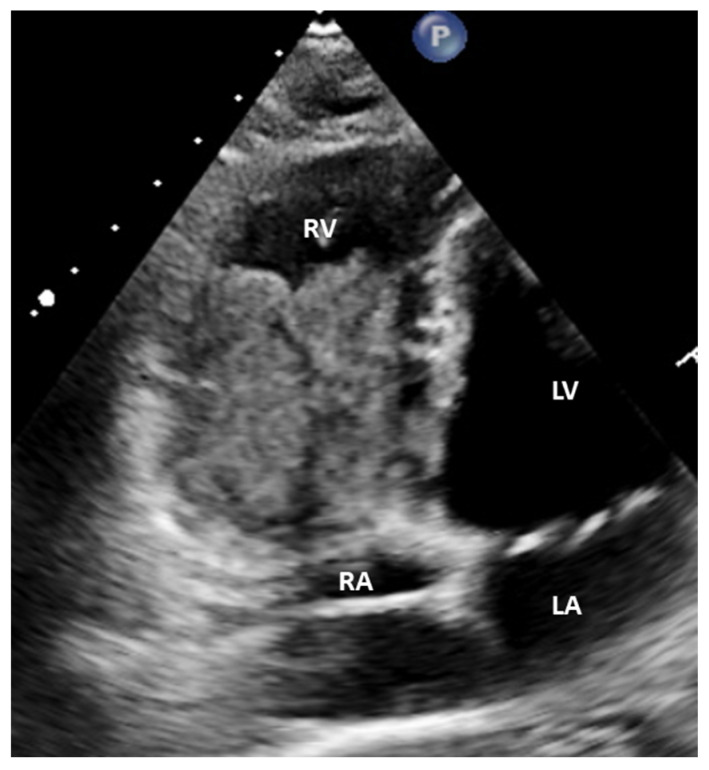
Echocardiogram, modified four chamber view: The tumor in RV has been enlarged occupying the majority of the chamber.
